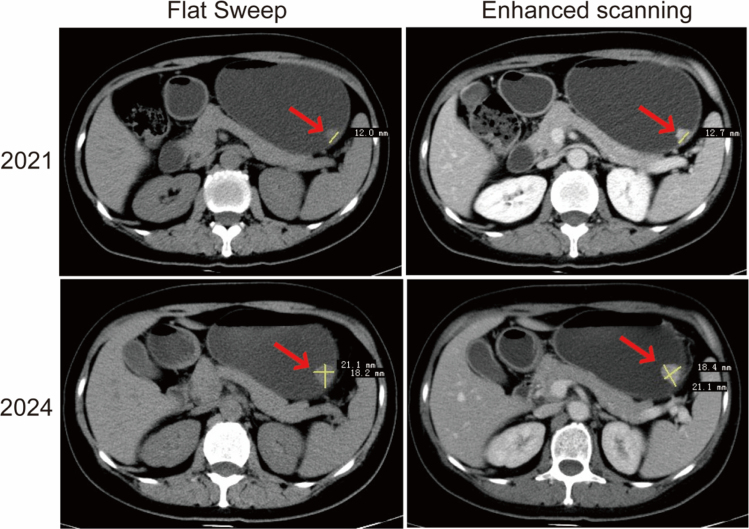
Computed tomography images from 2021 to 2024.
